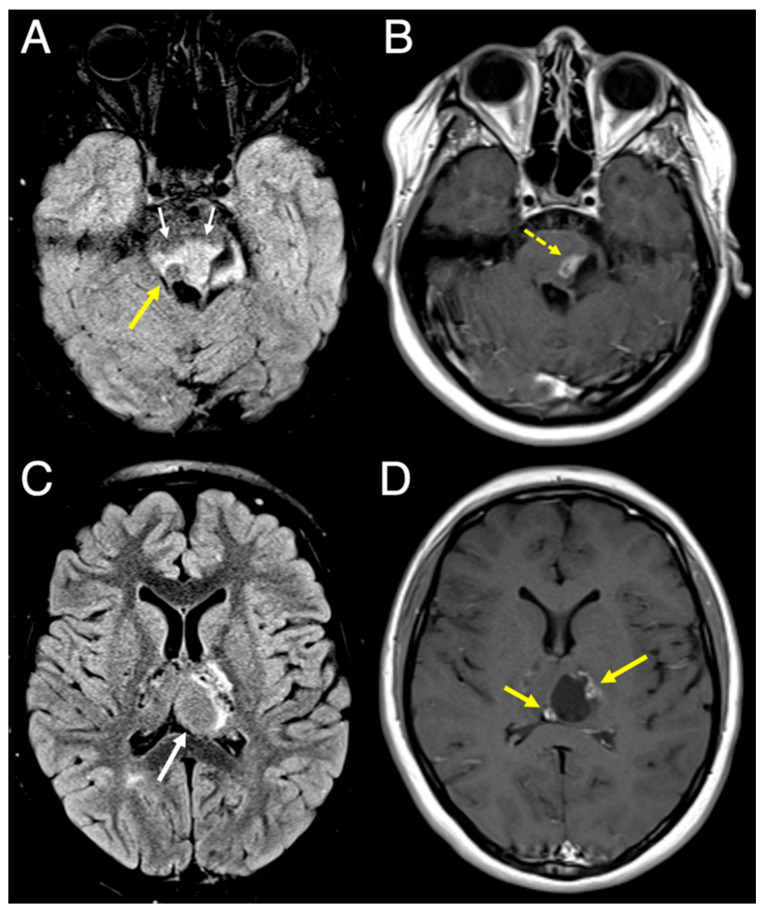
Five years after surgery, (A) fat-saturated FLAIR and (B) contrast-enhanced T1-TSE images show interval development of a new cyst (yellow arrow) in the right posterolateral aspect of the brainstem with surrounding abnormal white matter signal (white arrows) and worsening enhancement adjacent to prior residual cyst (dashed arrow) in the left aspect of the brainstem. (C) Fat-saturated FLAIR and (D) contrast-enhanced T1-TSE images at the basal ganglia level show new proteinaceous cyst formation (white arrow) in the left thalamus with surrounding linear and nodular enhancement (yellow arrows).
